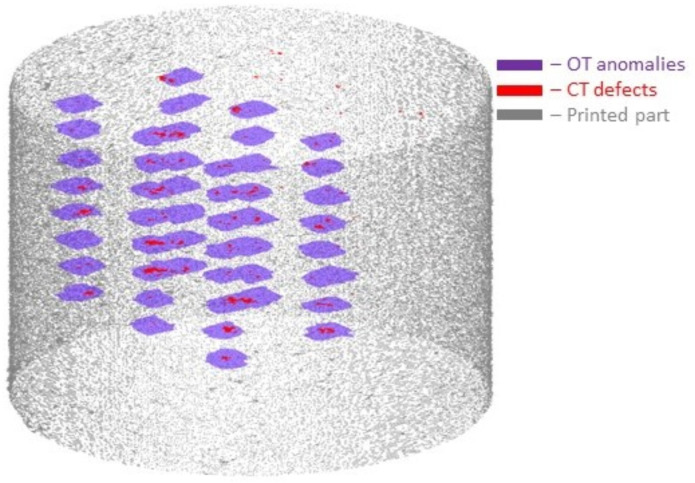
3-Dimensional rendered surface with overlap of CT defects with detected anomalies.
